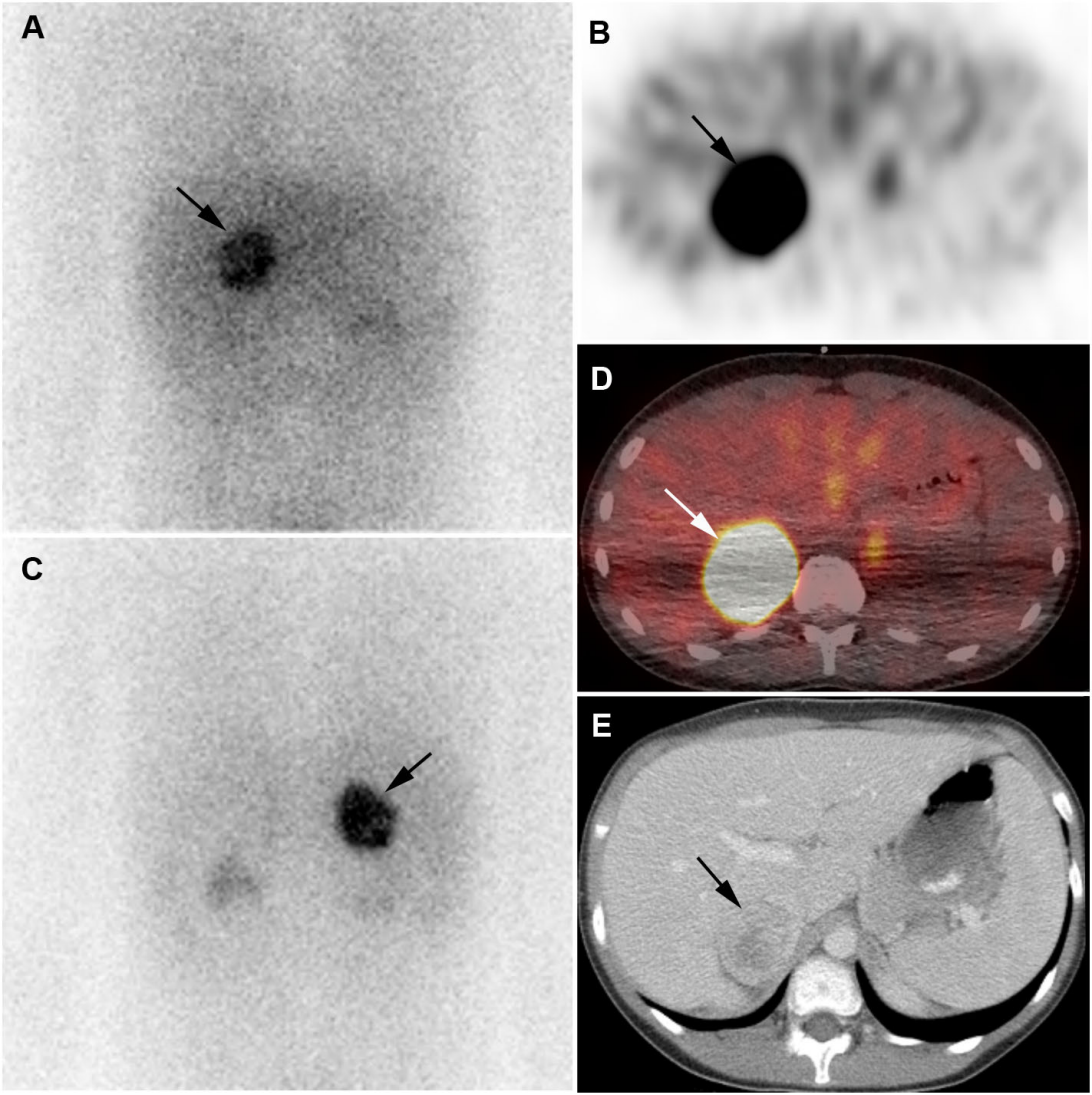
Pheochromocytoma. 11-year-old boy with pheochromocytoma. [^123^I]I-mIBG anterior planar image (A), transverse [^123^I]I-mIBG SPECT (B), [^123^I]I-mIBG posterior planar image (C), Fusion [^123^I]I-mIBG SPECT/CT (D), Diagnostic CT (E). Intense uptake of [^123^I]I-mIBG in right adrenal mass (arrows).
